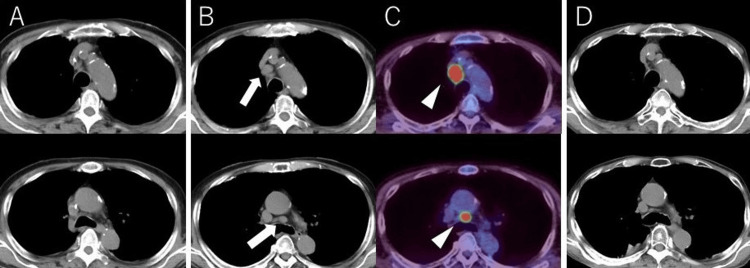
Axial images of serial unenhanced chest CT scans and axial PET-CT fusion. Chest CT scans on admission (A) showed no abnormalities. Follow-up chest CT scans soon after discharge showed enlarged mediastinal lymph nodes (B, arrows). One month after discharge, fluorodeoxyglucose PET-CT fusion showed increased uptake in the same region (C, arrowheads). After the chemoradiotherapy, the enlarged lymph nodes significantly reduced in size (D). CT, computed tomography; PET, positron emission tomography.
